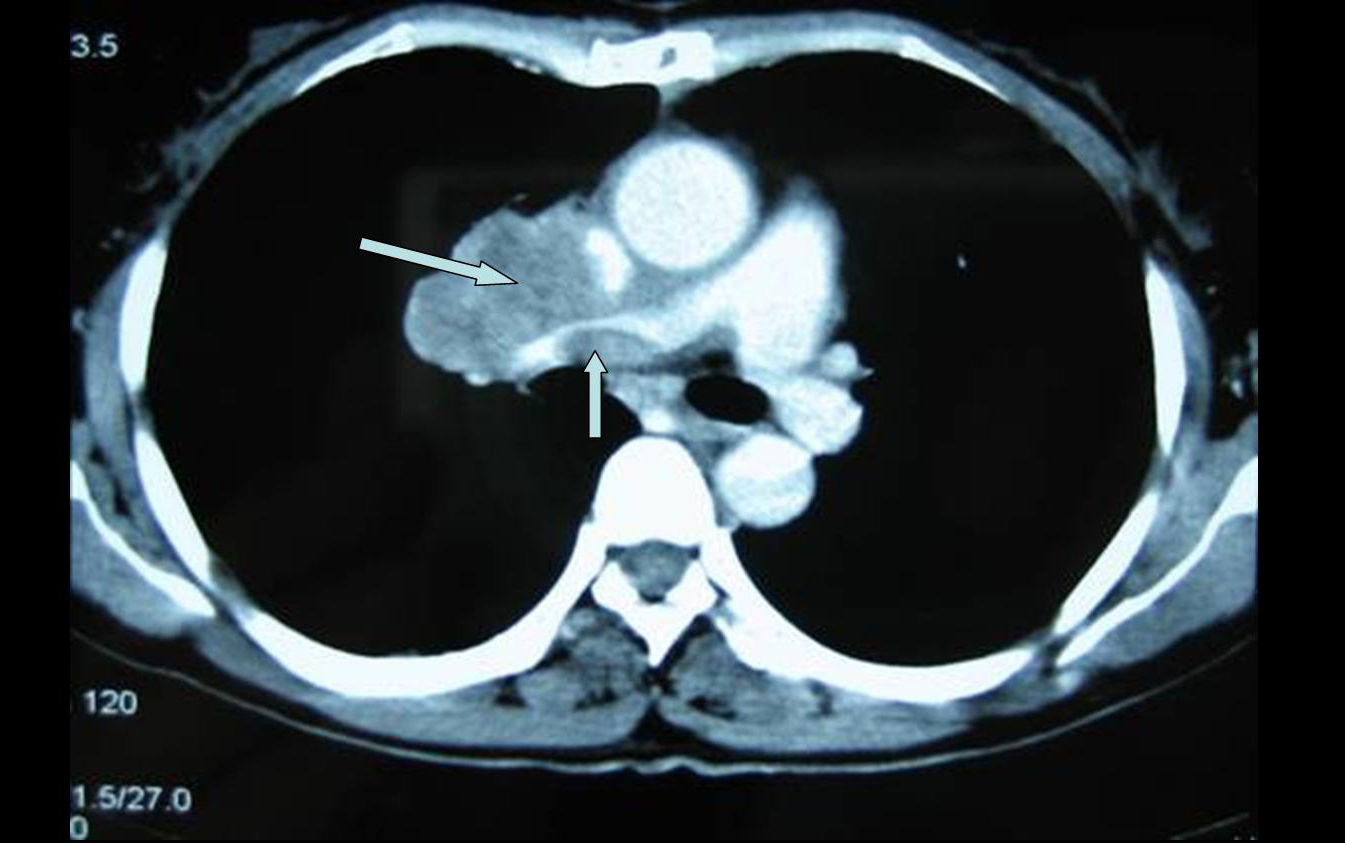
A computed tomography scan of the chest shows the recurrence of pulmonary artery intimal sarcoma.
